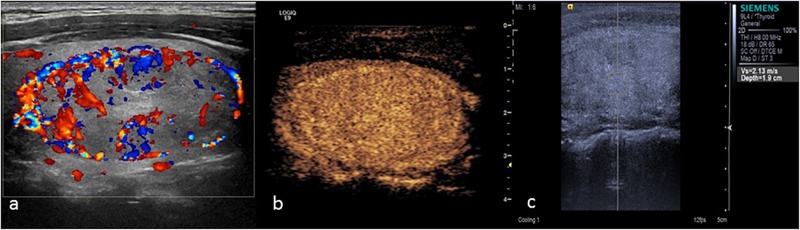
Papillary thyroid carcinoma. (a) Color Doppler ultrasound showing rich blood flow in the nodule. (b) CEUS showing heterogeneous homogeneous equal enhancement. (c) The SWV value was 1.53 m/s.
